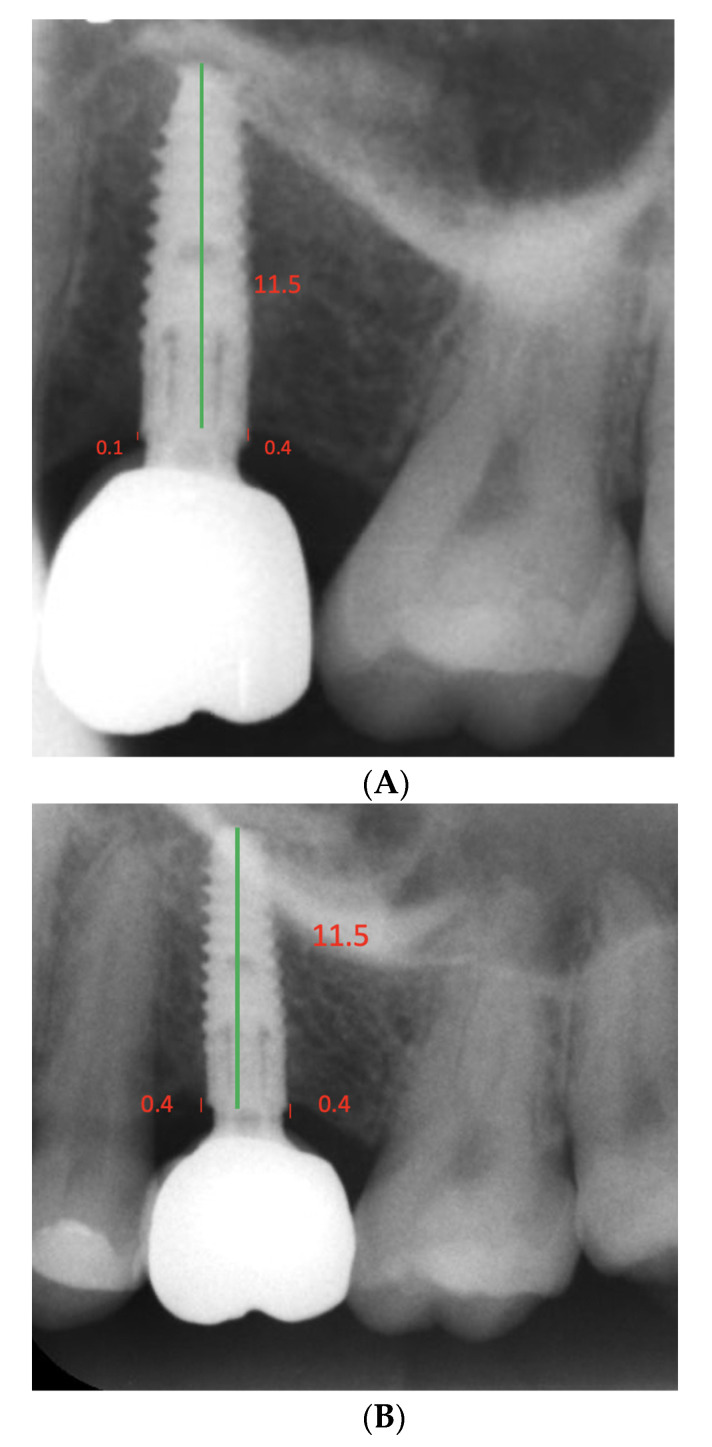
(A): Measurements of the hard tissue parameters using standardized long cone radiographs implant length (green vertical line). (B): Distance between the neck and the first bone-implant contact (red vertical line).
